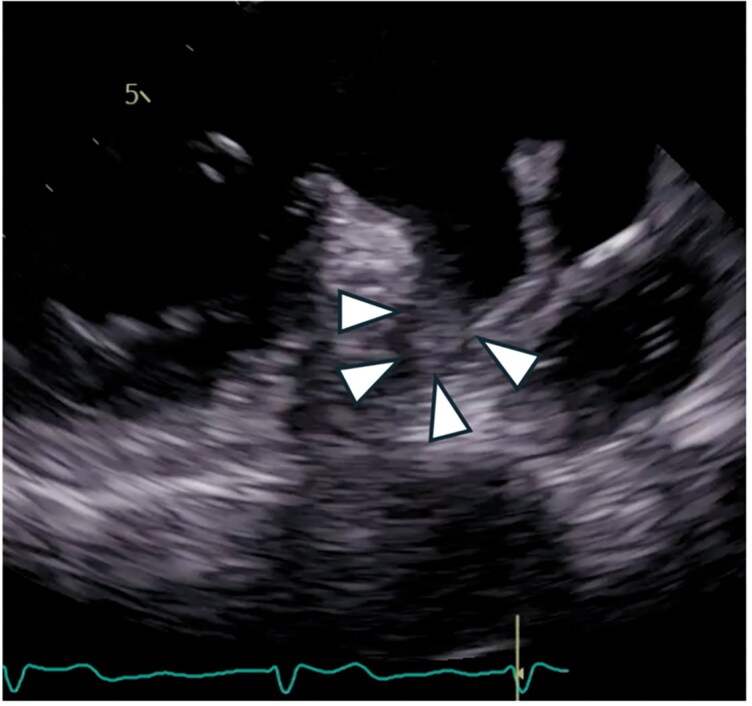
Pre-procedural transoesophageal echocardiography depicting left atrial appendage thrombus (triangles).
